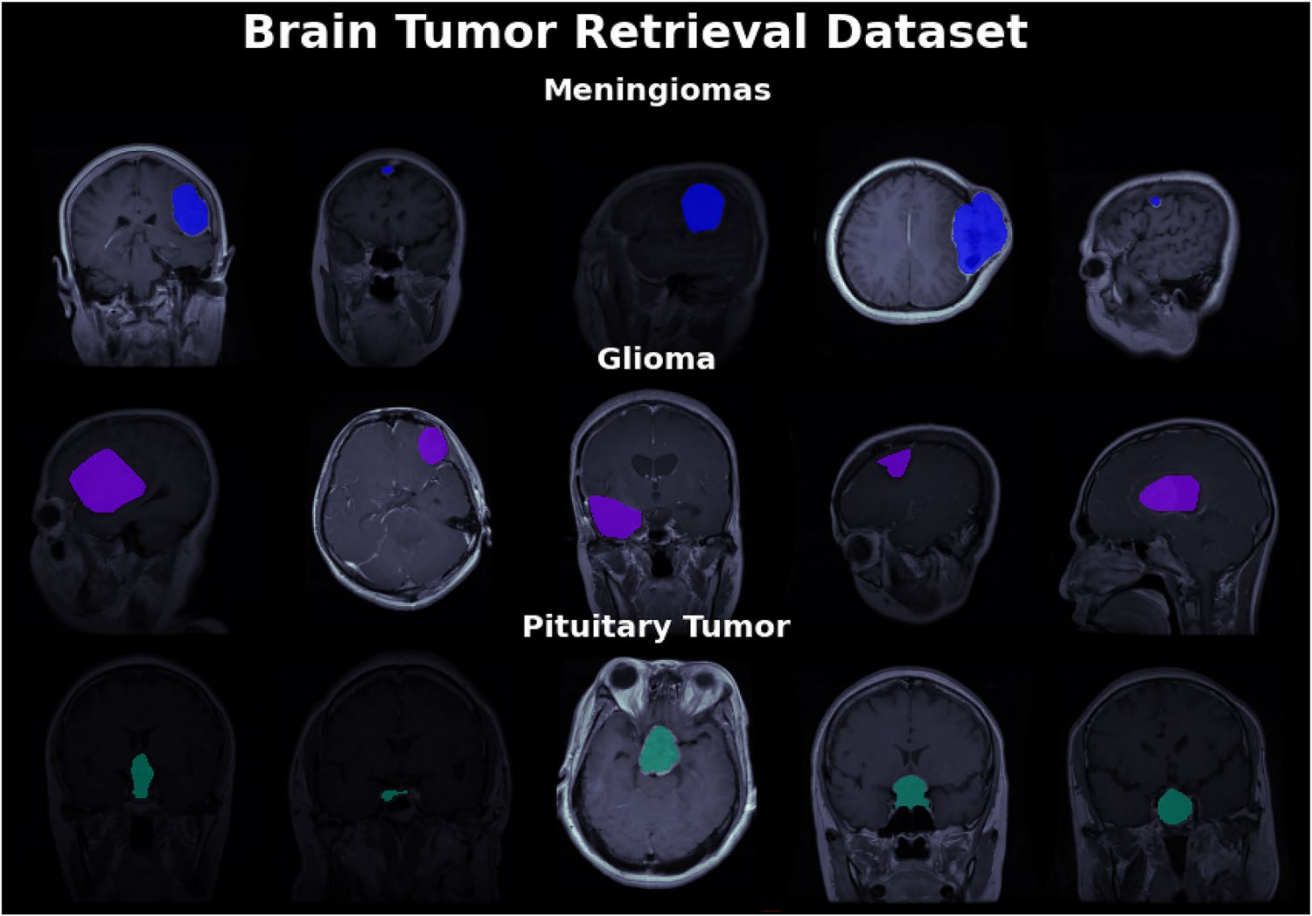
MR images of all tumor classes along with their tumor mask
